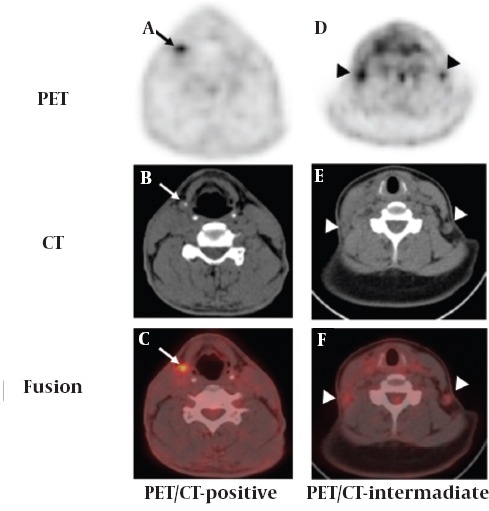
Representative cases of F-18 fluorodeoxyglucose (FDG) positron emission tomography/computed tomography (PET/CT). A PET/CT-positive case (A-C) involves a 48-year-old male showing a focal FDG uptake in the right cervical lymph nodes with the maximum SUV of 5.6 on PET (black arrow in A) and a malignant shaped LN, i.e. spherical LNs with eccentric calcification, suggesting metastasis on CT (white arrow in B). A PET/CT-intermediate case (D-F) involves a 47-year-old female showing a focal FDG uptake in both cervical LNs with the maximum SUV of 3.4 (left) and 3.8 (right) on PET (black arrowhead in D) and a benign shaped LN, i.e. an intact fatty hilum concaving into the central portion of LNs on CT (white arrowhead in E)
